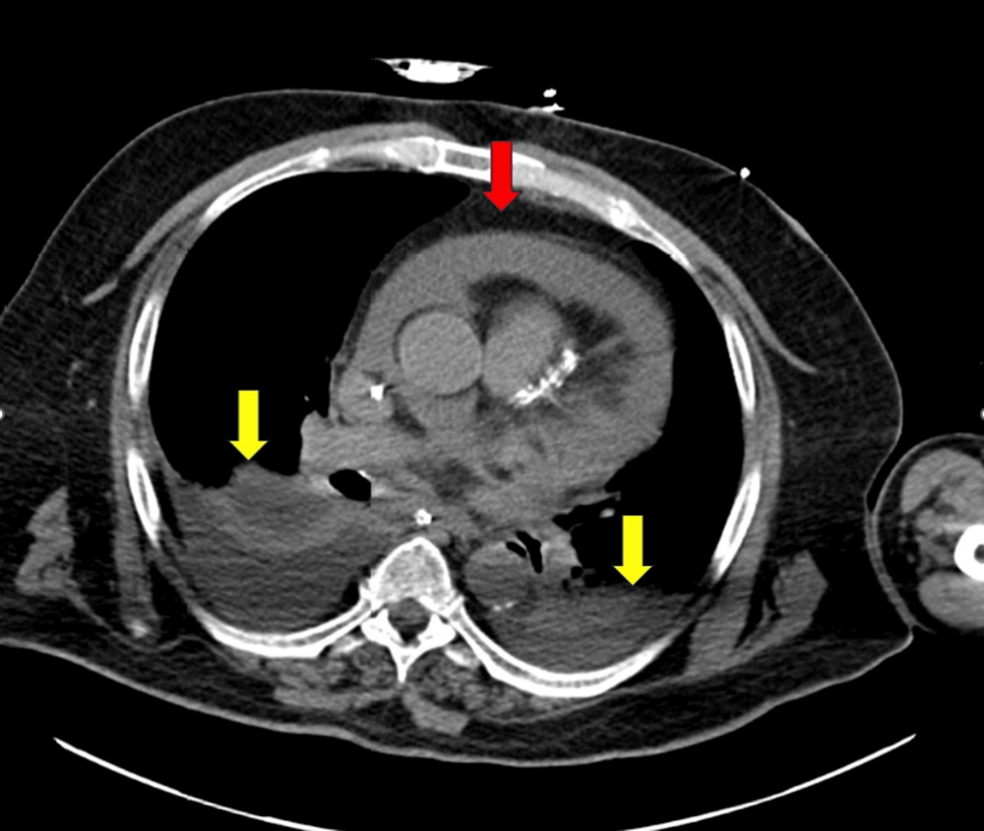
CT chest demonstrating pericardial effusion (red arrow), bilateral pleural effusions (yellow arrows)
